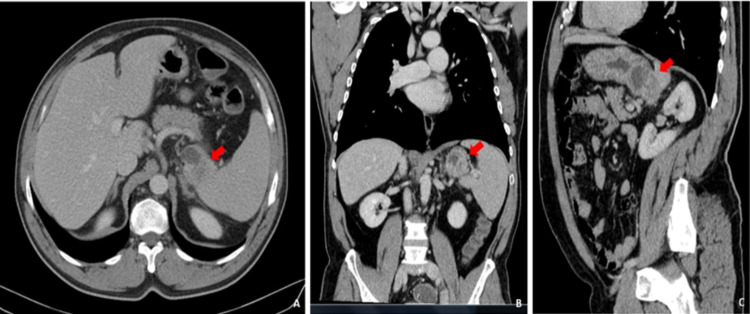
(A) axial, (B) coronal and (C) sagittal CT scans of the chest, abdomen and pelvis demonstrated a mixed mass with posterior tissue component and an anterior component of a cystic nature, localized in the greater gastric curvature, in contact with adjacent structures.
